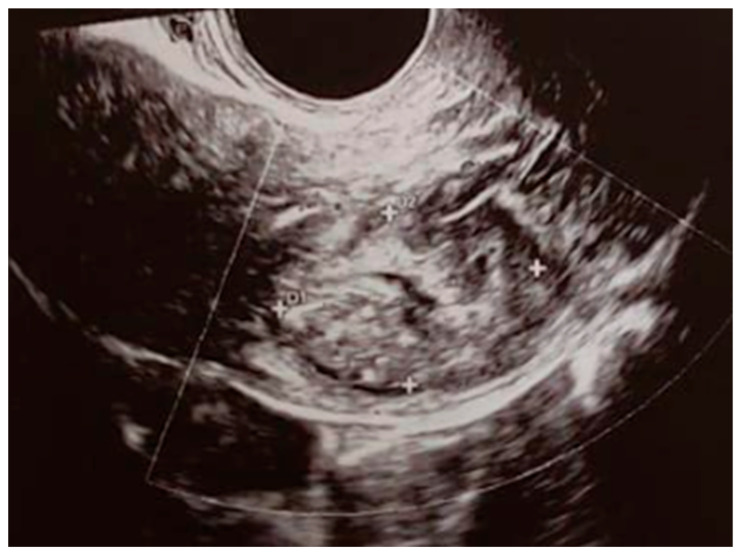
Ultrasound image taken 17 days post-treatment.
